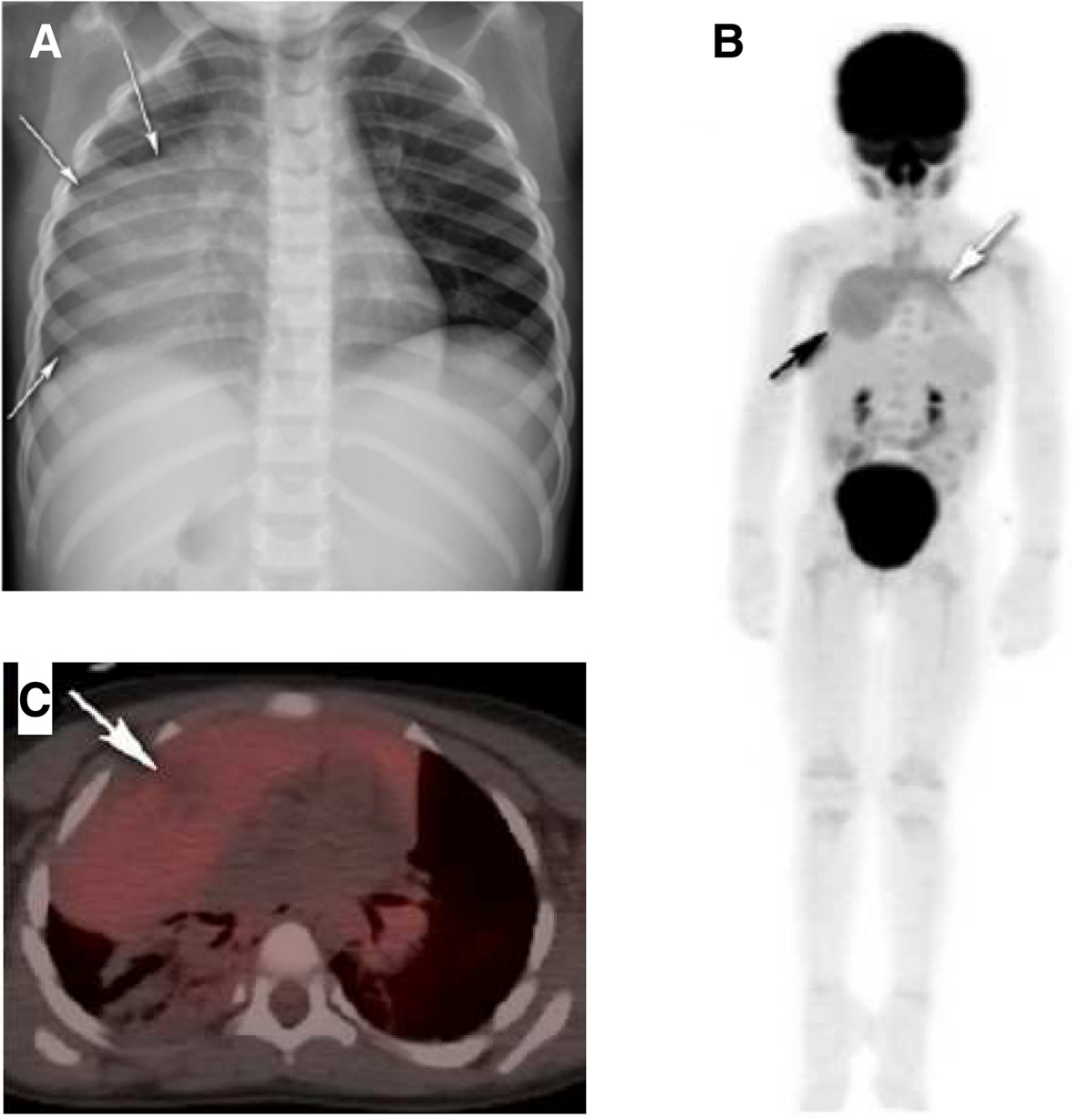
Diagnostic imaging studies of the presented patient. (A) Anteroposterior chest radiograph reveals a large predominately right-sided mass. (B) PET image shows that activity within the mass (black arrow) is indistinguishable from that in the normal thymus (white arrow). (C) Fused transaxial ^18^ F-FDG PET/CT shows only low-intensity, homogeneous ^18^ F-FDG avidity within the mass, with photopenia corresponding to the cystic/necrotic area (white arrow).
